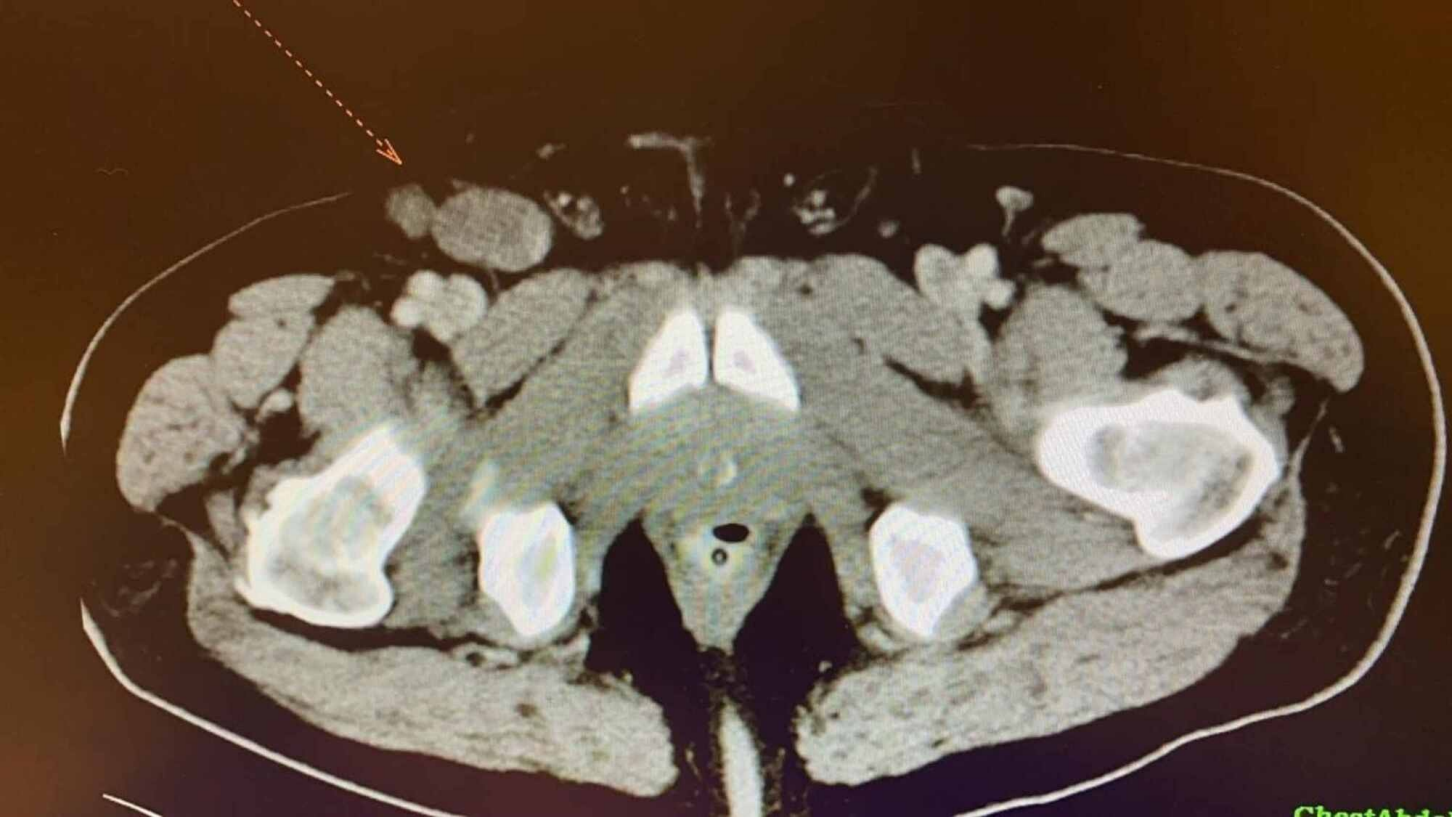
A cross-sectional slice of CT abdomen showing enlarged right inguinal lymph node.
